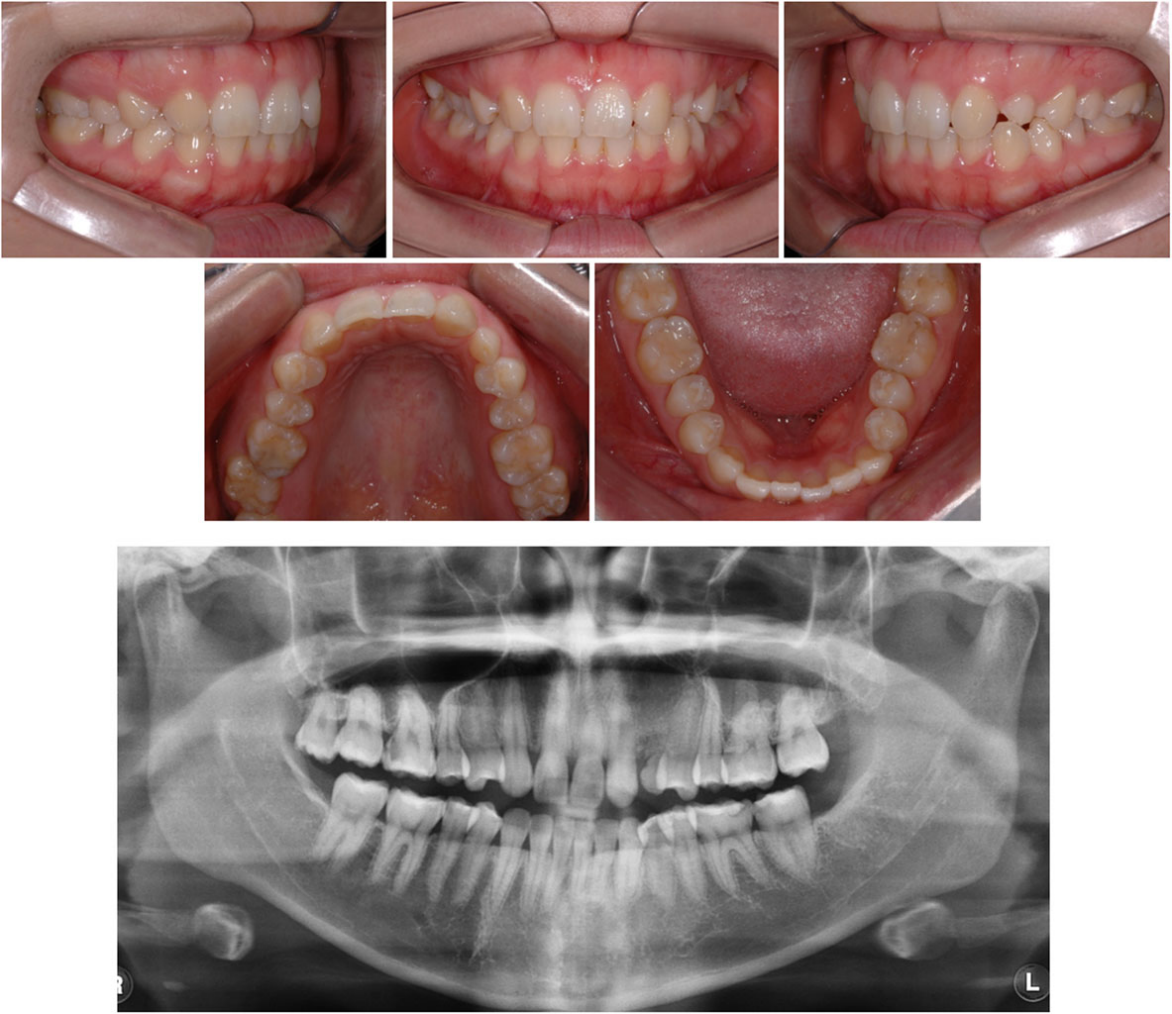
Pretreatment intraoral photographs and panoramic radiograph
